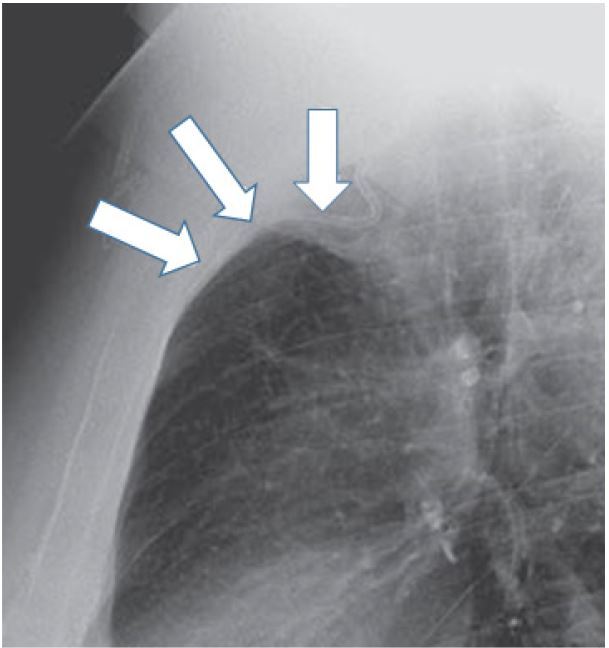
Lateral chest radiograph of patient in Figure 5 showing catheter (arrows) malposition coursing anteriorly along right internal mammary vein.
